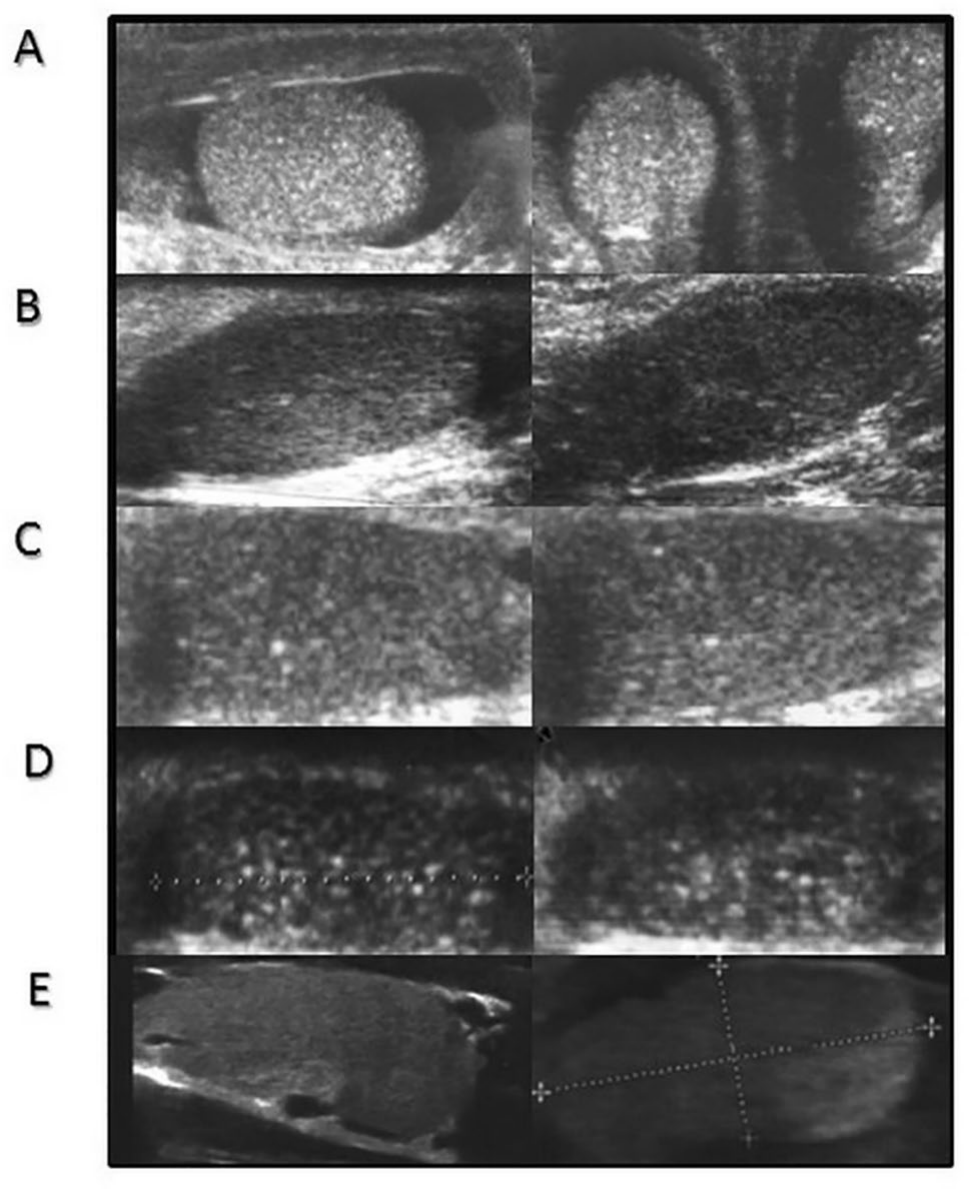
Ultrasound features of testes with TM in the infants from the mini-puberty group. A 3 months old, B 4 months old, C and D 5 months old, E healthy control 5 months old
